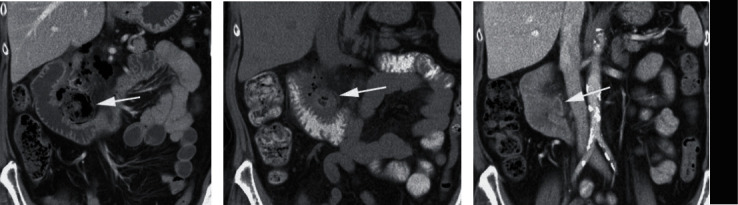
Duodenal diverticulitis. Axial CT scan shows inflamed diverticulum (arrow) medial to the duodenum (arrowheads). There was also free retroperitoneal air (not shown here).
